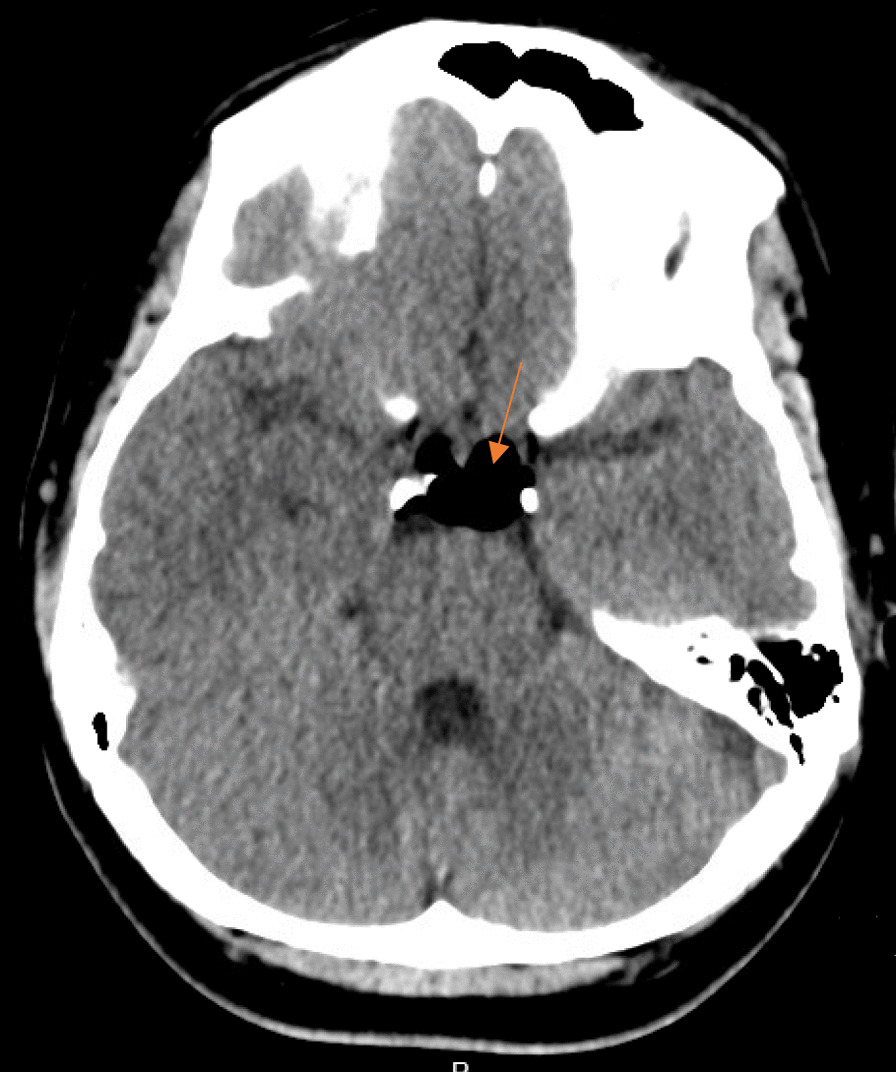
Computed tomography head showing air within the suprasellar cistern. Orange arrows identify the abnormal presence of air
